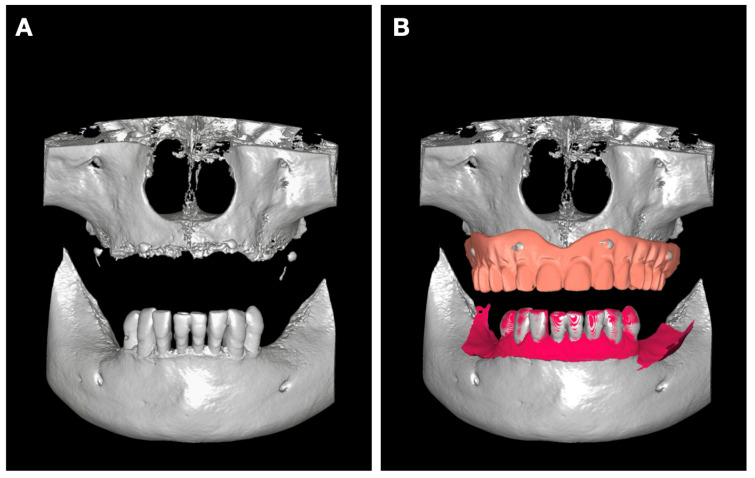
(A) 3D reconstruction from CBCT; (B) overlay of intraoral scans on the CBCT reconstruction. The maxillary prosthesis was overlaid using radiopaque markers, while the lower arch scan was aligned using dental units as references.
